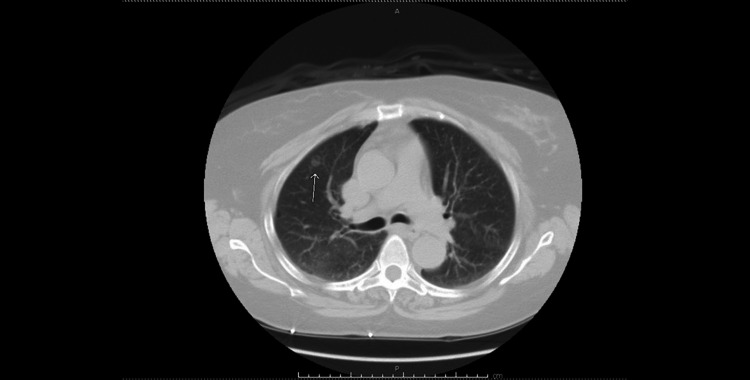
CT chest without contrast. White arrow showing right upper lobe nodule that was biopsied.
